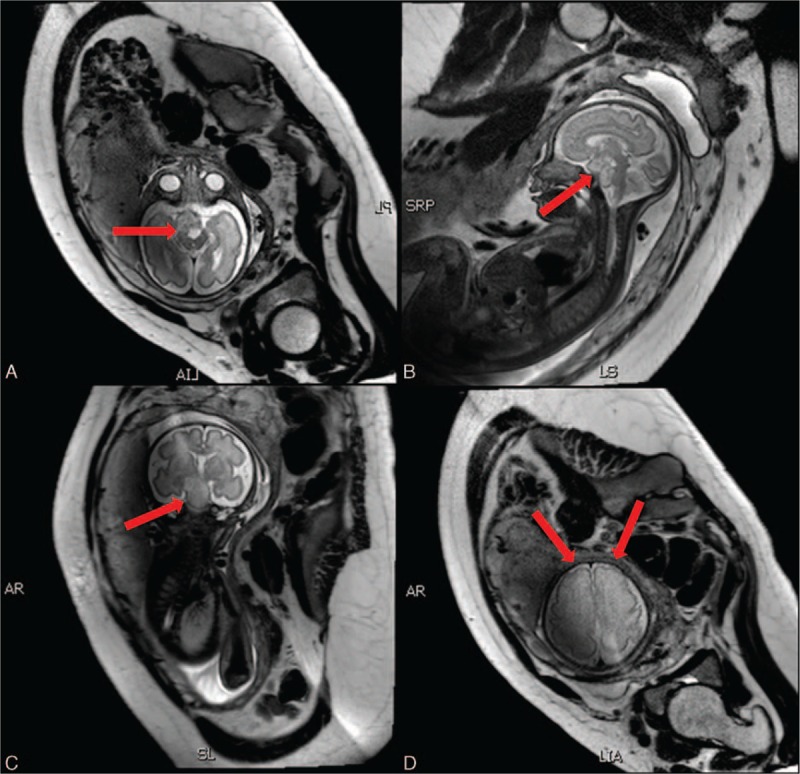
Fetal intracranial tumor was shown (red arrowhead) by axial view (A), sagittal view (B) and coronal view (C) of MRI. Fetal brain dysplasia (D) was diagnosed by the poor developed gyri and sulci of the frontal lobe (red arrowhead). MRI = magnetic resonance imaging.
